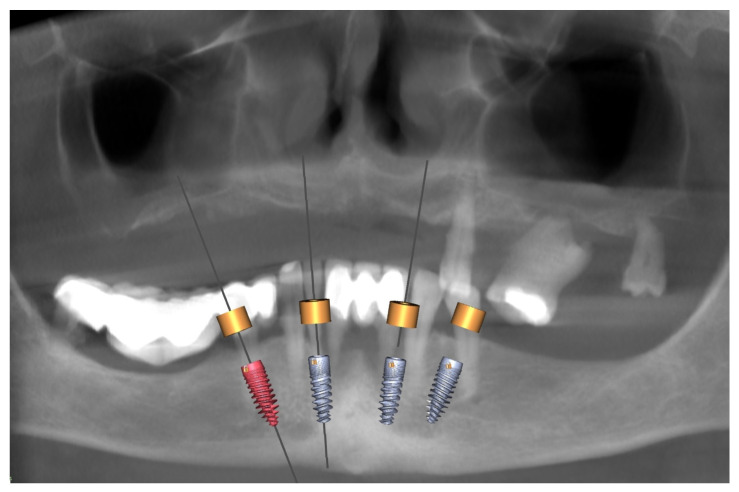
Planning for the mandible. An OPG image was obtained using CBCT. The planned positions of four implants were obtained using Blue Sky Bio software.
